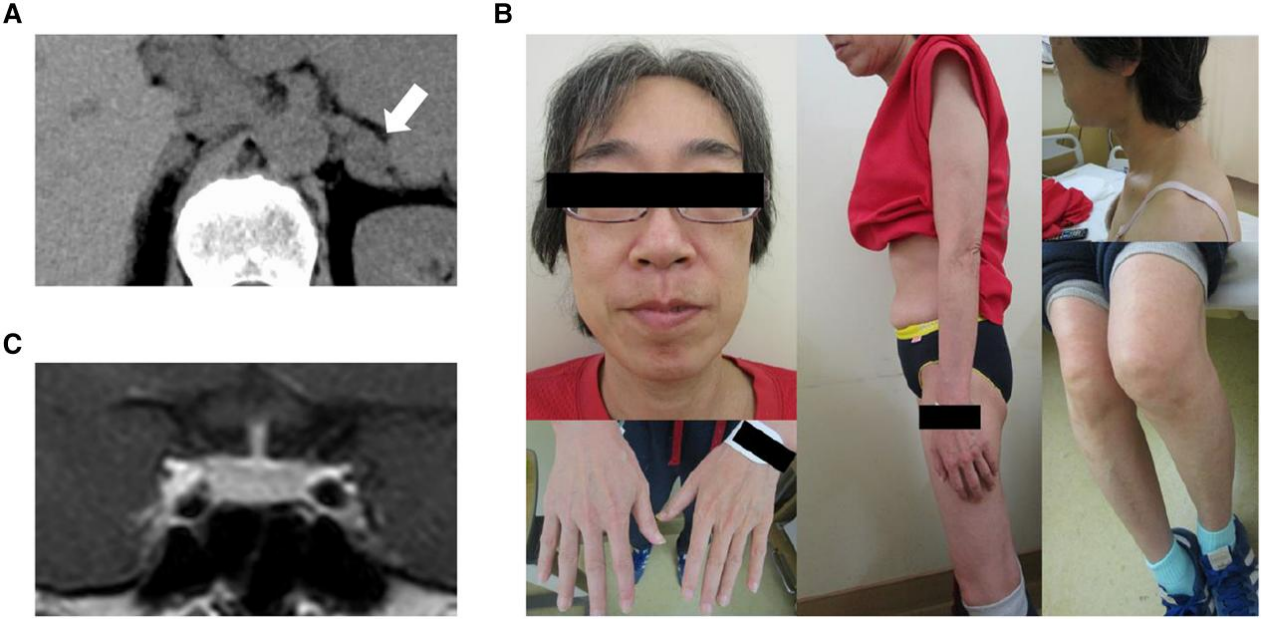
Clinical findings on patient imaging and physical examination. (A) Findings of abdominal CT scan. The white arrow indicates the left adrenal tumor, which is 20 mm in size and has a CT value of 10 HU. (B) Photo of the patient. No signs suggestive of Cushingoid symptoms, including moon face, central obesity, purple striae, skin atrophy, proximal myopathy, peripheral edema, or hirsutism, are observed. (C) MRI of the pituitary gland with a contrast reagent. Abbreviations: CT, computed tomography; HU, Hounsfield units; MRI, magnetic resonance imaging.
